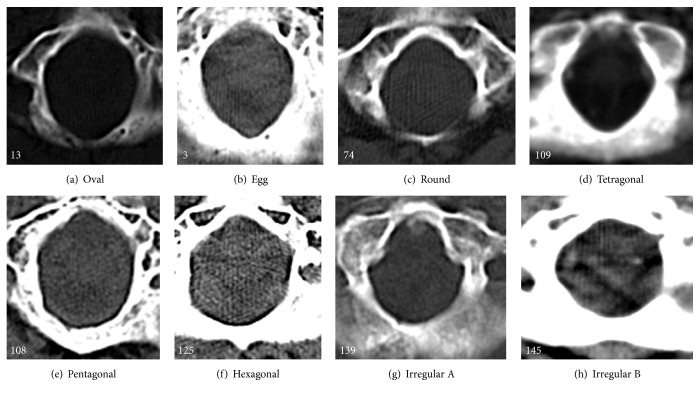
Different shapes of foramen magnum as studied in cranial CT scans.
